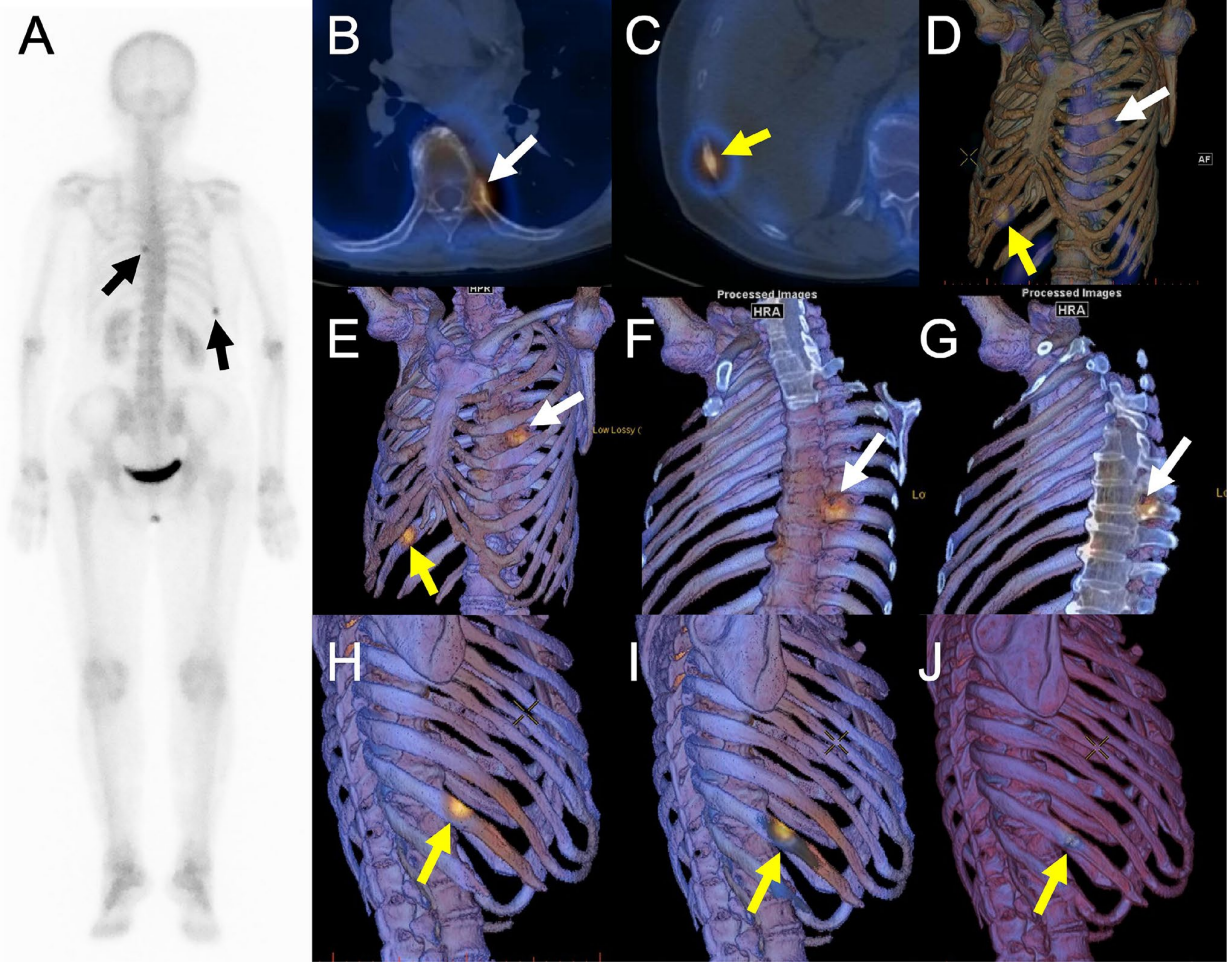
Whole-body scintigraphy (a), 2D SPECT/CT fusion (b and c), 3D SPECT/CT fusion using volume-rendered SPECT images (d) or image data projection of bone SPECT onto 3D volume-rendered CT images without (e, h, and j) or with clip-plane editing (f, g, and i) in a breast cancer patient with co-existence of bone metastasis and fracture in the ribs. Osteolytic change is clearly seen in 2D SPECT/CT (b) and our 3D SPECT/CT (f and g) images in the proximal site of the left eighth rib (*white arrows*). By contrast, a fracture line is shown at the hypermetabolic site in 3D SPECT/CT fusion (h–j)
